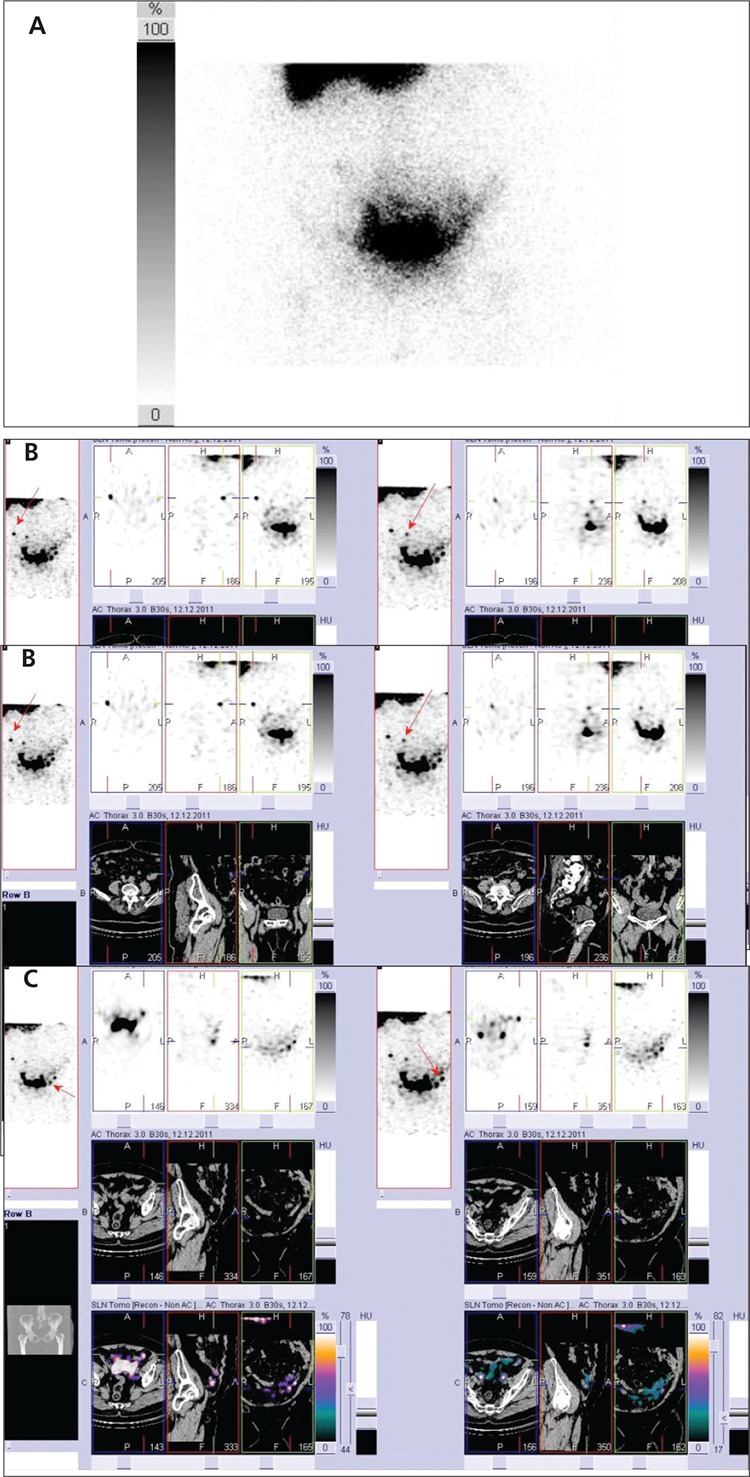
73 year old woman with endometrial cancer. Planar scintigram in anterior projection (A) shows only suspected SLN on the right without possibility to localize it. Fusion of SPECT/CT (B, C) shows two SLNs on the right(B) and two SLNs on the left side (C) of the pelvis
